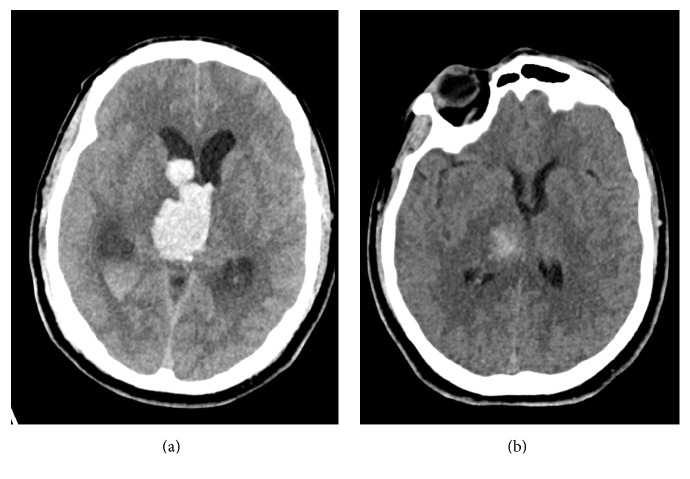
Computed tomography (CT) of the head. (a) CT head on admission showing a right thalamic hemorrhage with intraventricular extension. Note the obstructive hydrocephalus. (b) CT head on hospital day 9 showing evolution of the right thalamic hemorrhage and resolution of the obstructive hydrocephalus with external ventricular drainage (not shown) and after intraventricular tissue plasminogen activator (tPA).
